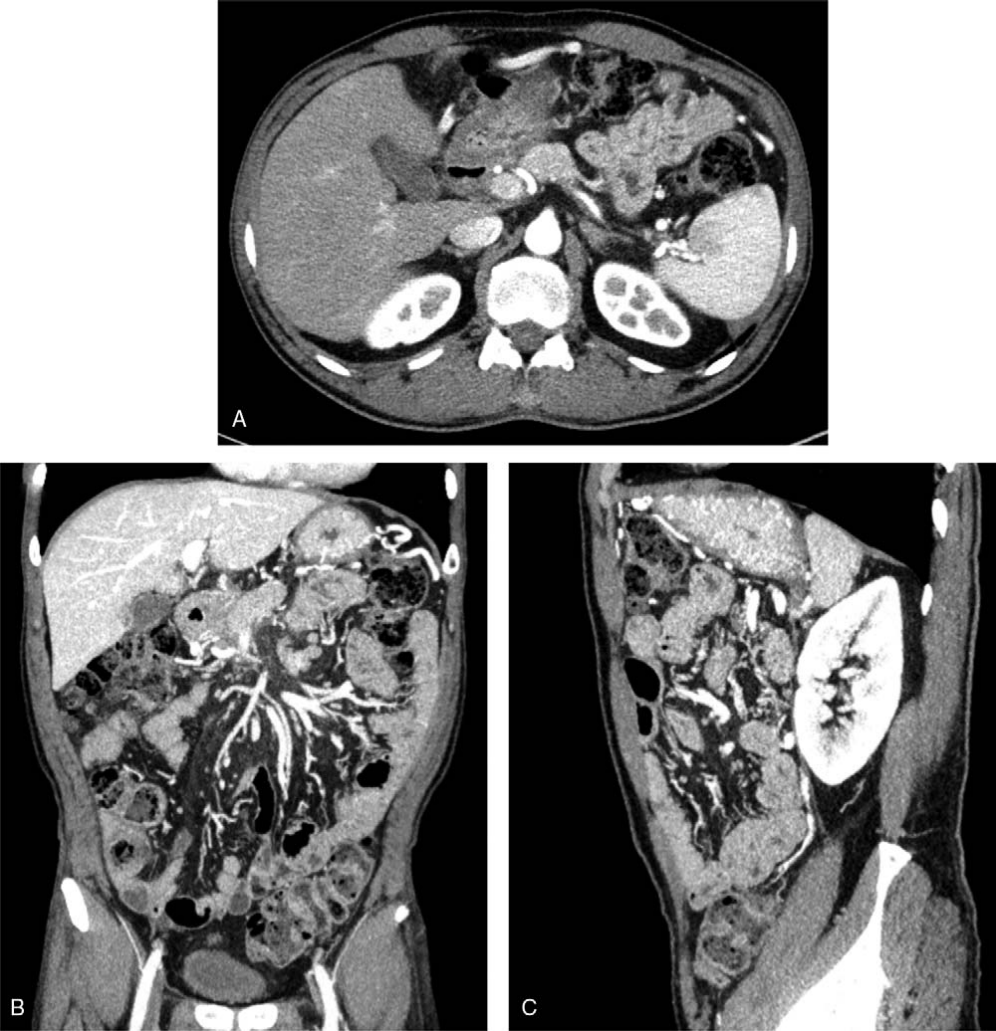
Abdominal CT showing the complete resolution of the emphysematous pancreatitis. A: An axial CT scan at the level of the pancreas body and tail. B: A coronal CT scan at the level of the pancreas head and mesenteric root. C: A sagittal CT scan at the level of the left anterior pararenal space. CT = computed tomography.
